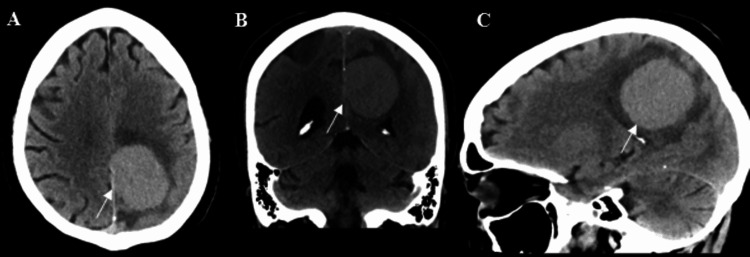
Initial CT Head imaging with axial (A), coronal (B), and sagittal (C) views. Imaging shows a hyperdense left frontoparietal mass along the falx cerebri with surrounding vasogenic edema.
